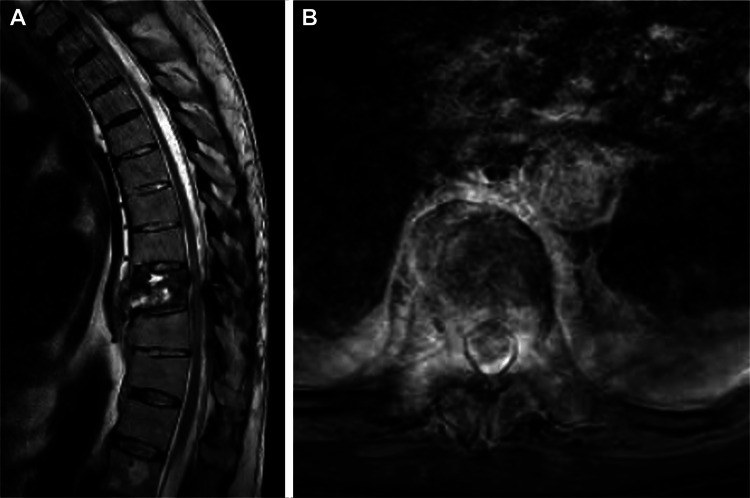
Preoperative MRI of the lumbar spine in case 2. Sagittal (A) and axial (B) imaging revealed spinal cord compression but no sign of spinal subdural hematoma. Used with permission from Barrow Neurological Institute, Phoenix, Arizona.
